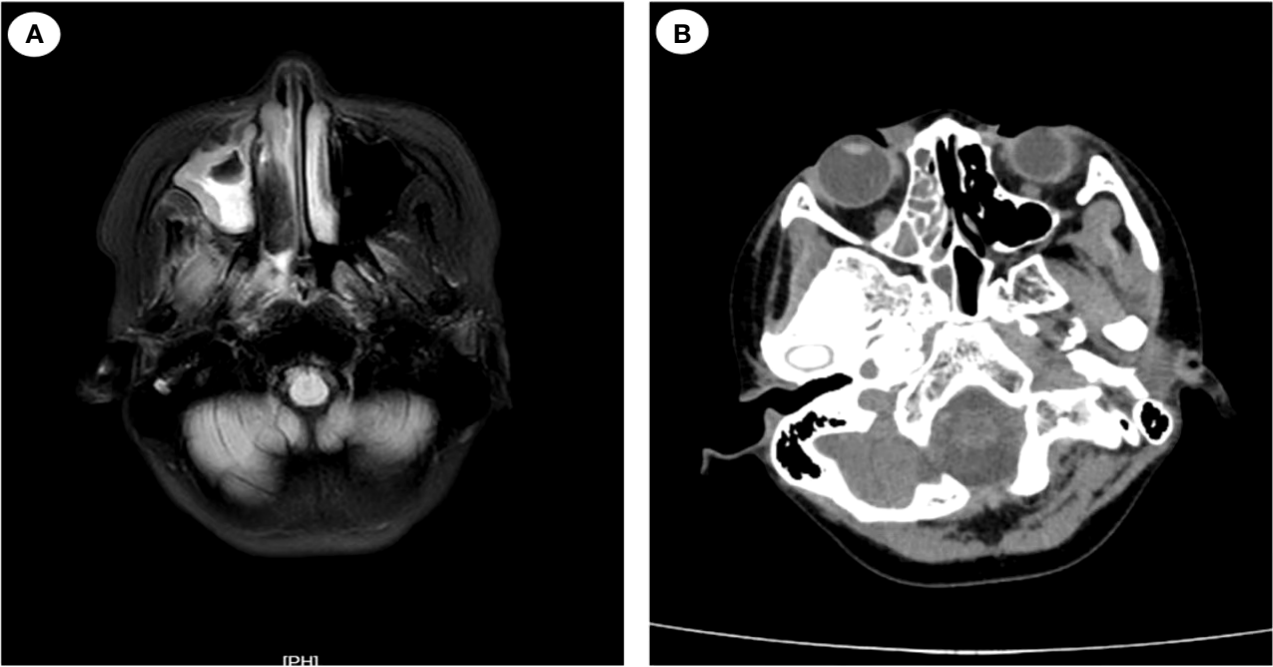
Magnetic resonance imaging (MRI) and computed tomography (CT) images of the patient’s head (case 1). (A) MRI of the patient’s head on July 17: abnormal signal shadow of the ethmoid sinus, sphenoid sinus, and bilateral maxillary sinus. (B) CT scans of nasopharynx on July 24: soft tissue shadows are observed in the right nasopharynx, ethmoid sinus, nasal cavity, and maxillary sinus.
